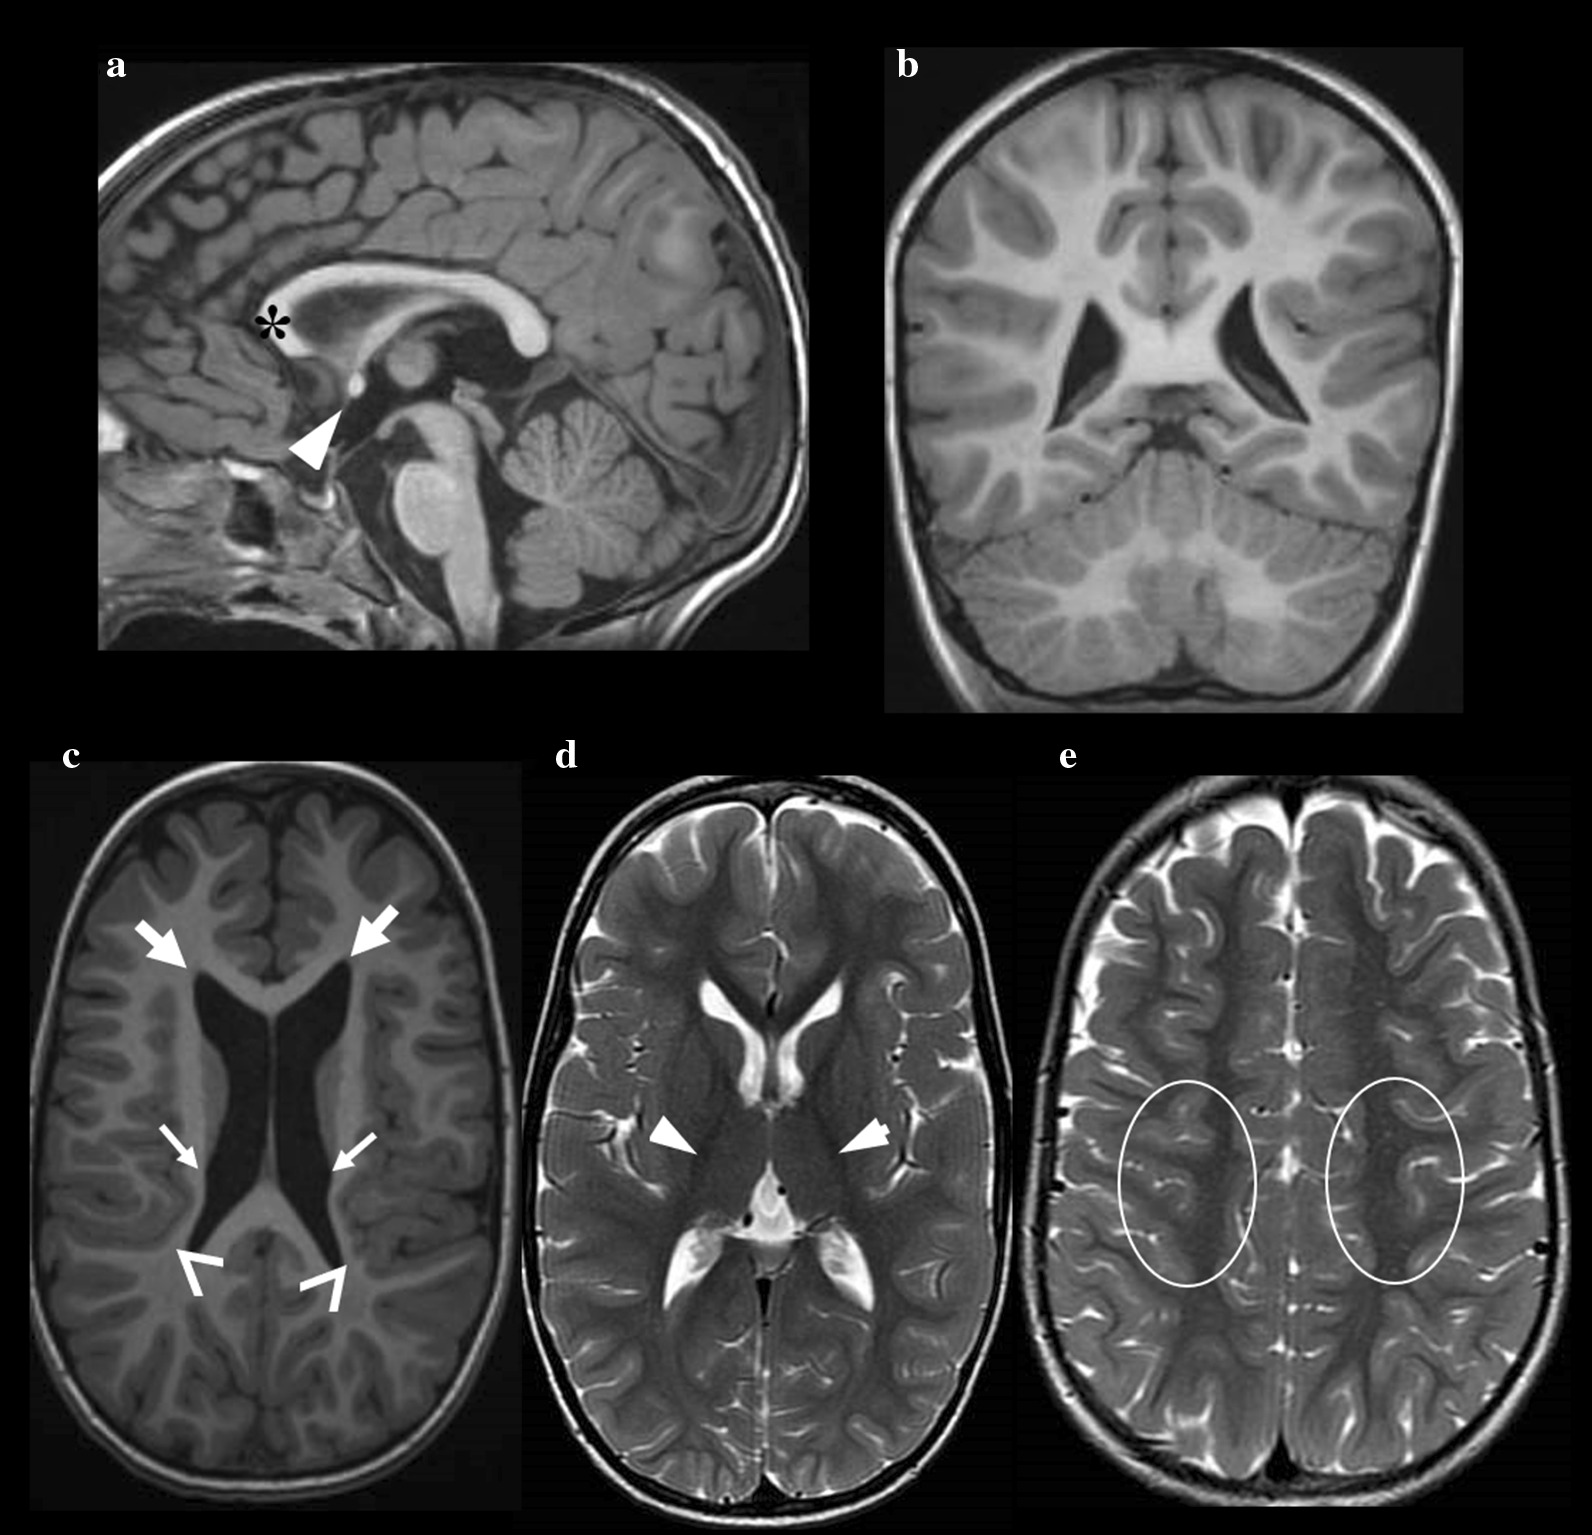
Magnetic Resonance Imaging scans of Patient-3. Sagittal T1-weighted image (a) shows a thinning of the genu of the corpus callosum (asterisk) and a slight hypetrophy of the anterior commissure (head-arrow). Scaphocephalic skull is also evident. Sagittal (a) and coronal (b) T1-weighted images show normal cerebellum. Axial T1-weighted image (c) shows dysmorphic features of lateral ventricles with dilatation of the frontal horns (arrow) and irregular ventricular walls (small arrows). A reduction of volume of insulo-temporal white matter (head-arrow) is also evident. Axial T2-weighted images (d–e) show normal signal of the white matter at the level of the posterior limb of the internal capsule (head-arrow) and of the paracentral region (oval)
